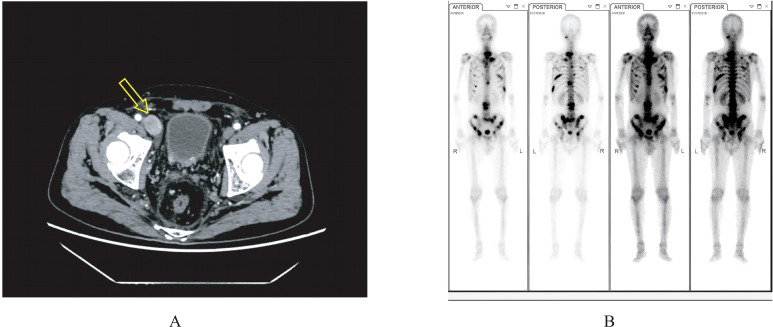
(A) Enhanced computed tomography (CT) scan of the abdomen and pelvis, axial view, performed on July 15, 2024, demonstrating right inguinal lymphadenopathy (yellow arrow). (B) Anterior and posterior whole-body skeletal scintigraphy with 99mTc-methylene diphosphonate (MDP), performed on July 22, 2024, showing multiple foci of increased radiotracer uptake consistent with bone metastases.
